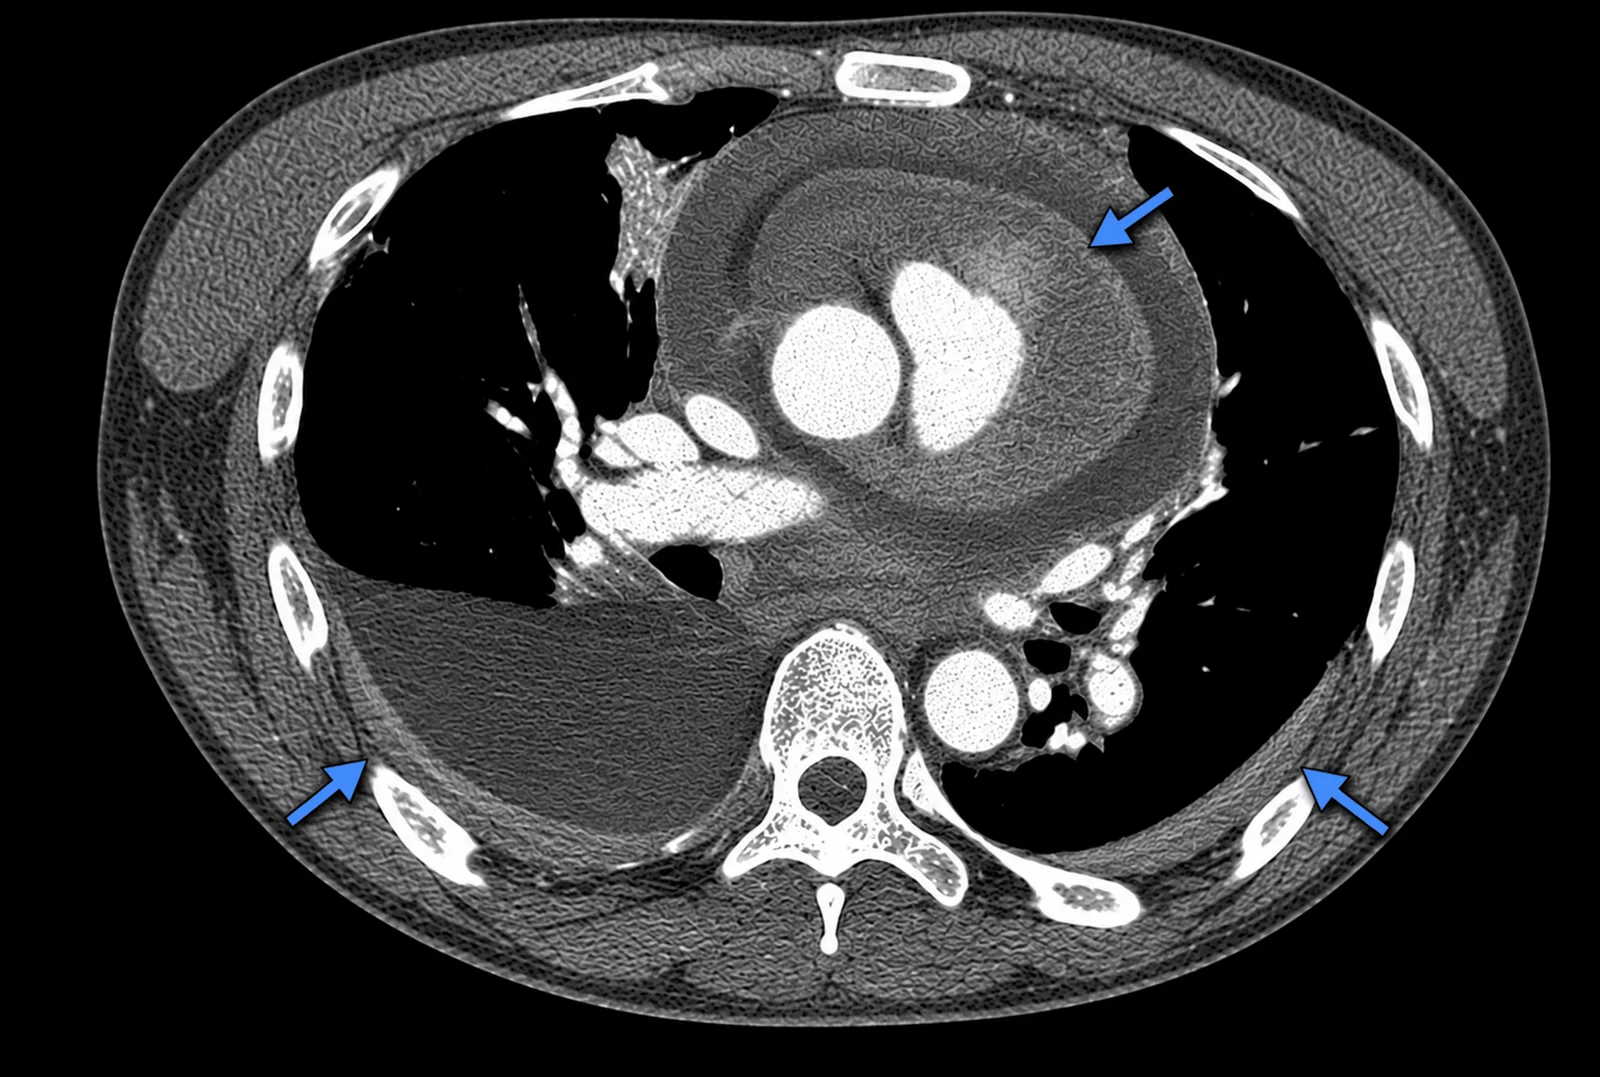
Computed tomography angiography (CTA) demonstrating incidental cardiopulmonary findings. The upper arrow indicates a large pericardial effusion, while the lower arrows indicate bilateral pleural effusions, greater on the right. CTA showed no large-vessel occlusion or hemodynamically significant stenosis. The pericardial effusion was incompletely imaged on this examination.
